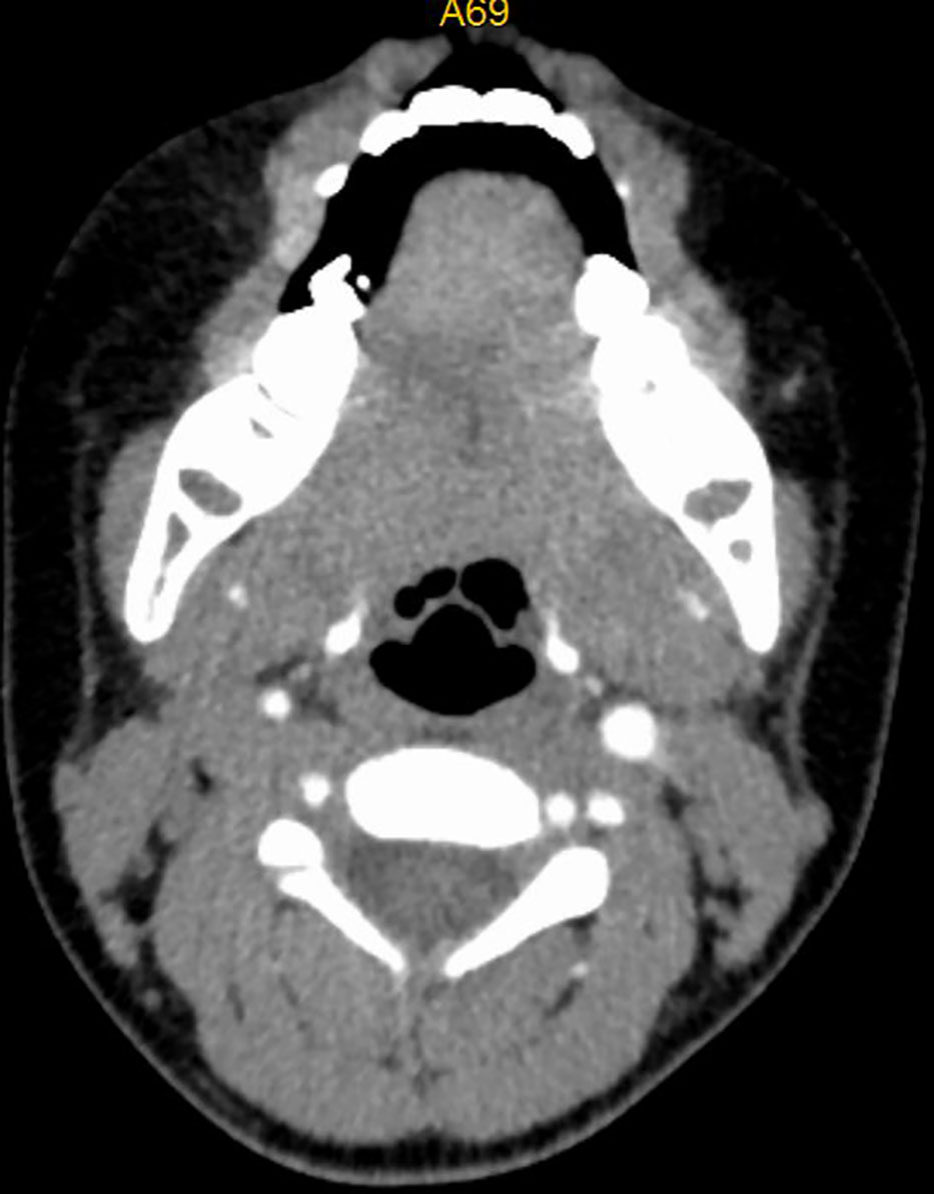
CT image of No. 8 patient after embolization treatment, hemangioma disappeared, no airway stenosis.
